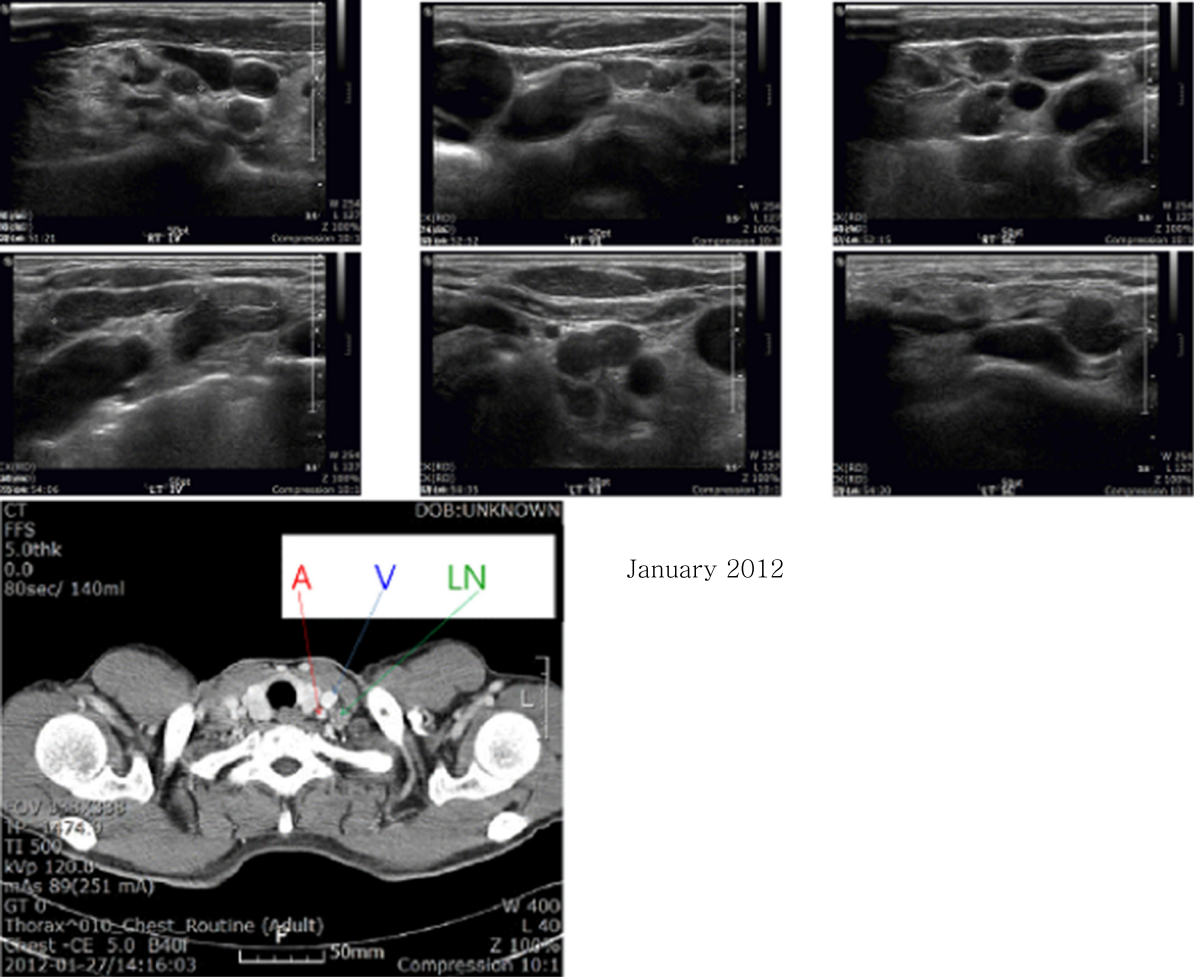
Thyroid and neck sonography. Enlargement of multiple lymph nodes appears like metastases at both neck levels IV and VI and the supraclavicular level. CT with enhancement. Lymph node enlargement about 2 cm in size at the left supraclavicular area. Abbreviation (arrows): A, common carotid artery. V, jugular vein. LN, supraclavicular lymph node.
